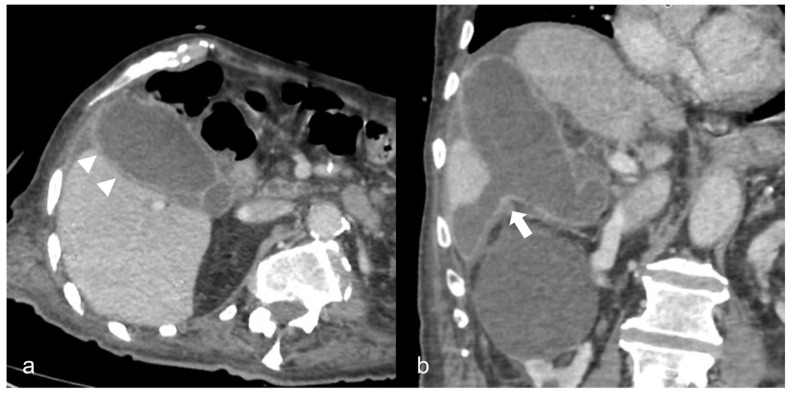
A 90-year-old COVID-19 patient presented acute right quadrant abdominal pain and cholestasis. Computed tomography on the axial (a) and coronal (b) plane showed acute cholecystitis with diffuse thickening of the gallbladder wall and pericholecystic fluid (arrowheads). Disruption of the gallbladder wall (arrow) is also present with fluid collection around the liver indicating perforation.
